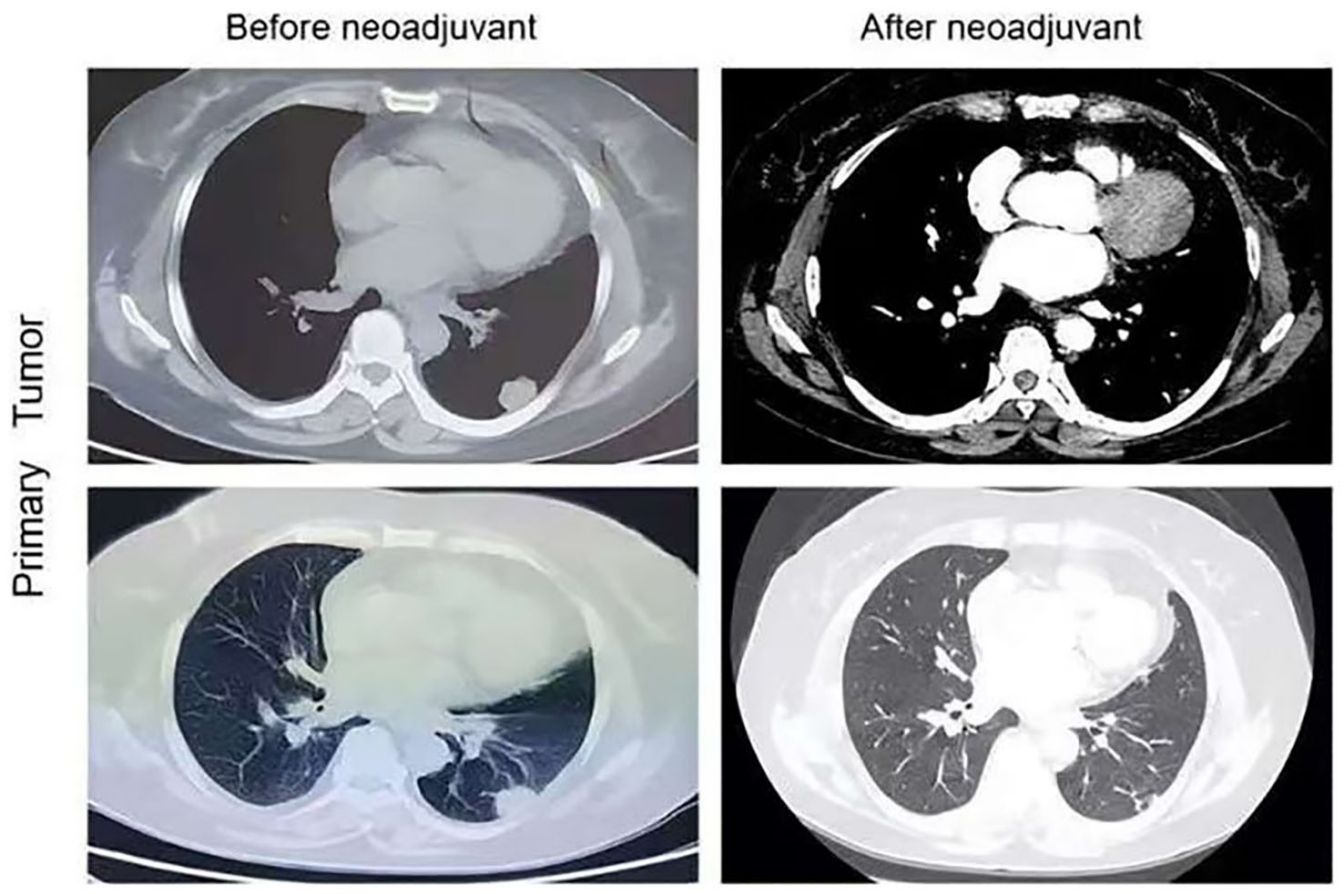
Chest CT images of left lung adenocarcinoma before and after the neoadjuvant therapy.
